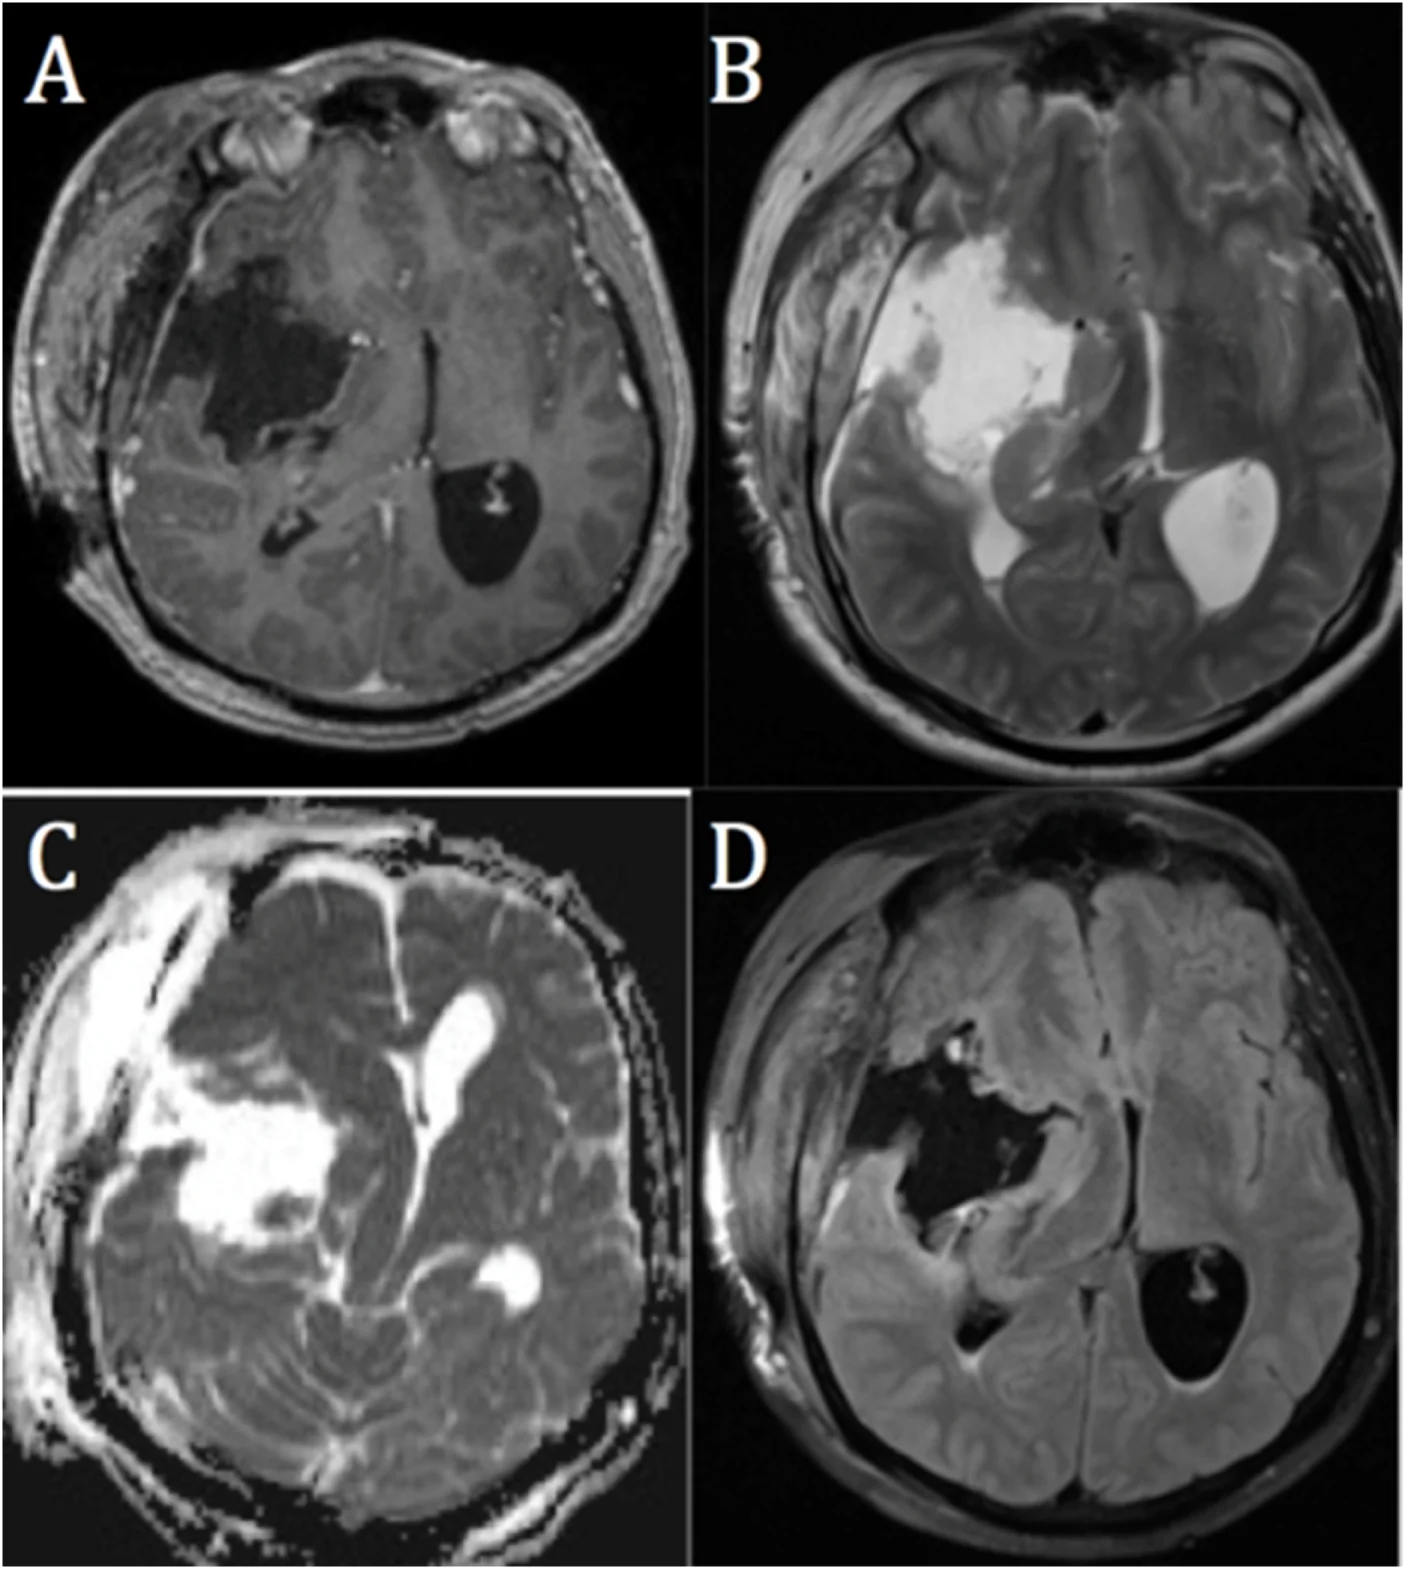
T1-weighted image (A), T2-weighted image (B), ADC (C), and FLAIR (D), showing near-complete resection postoperatively, with a small residual part shown on ADC (C) over the medial portion at the tumor location.
